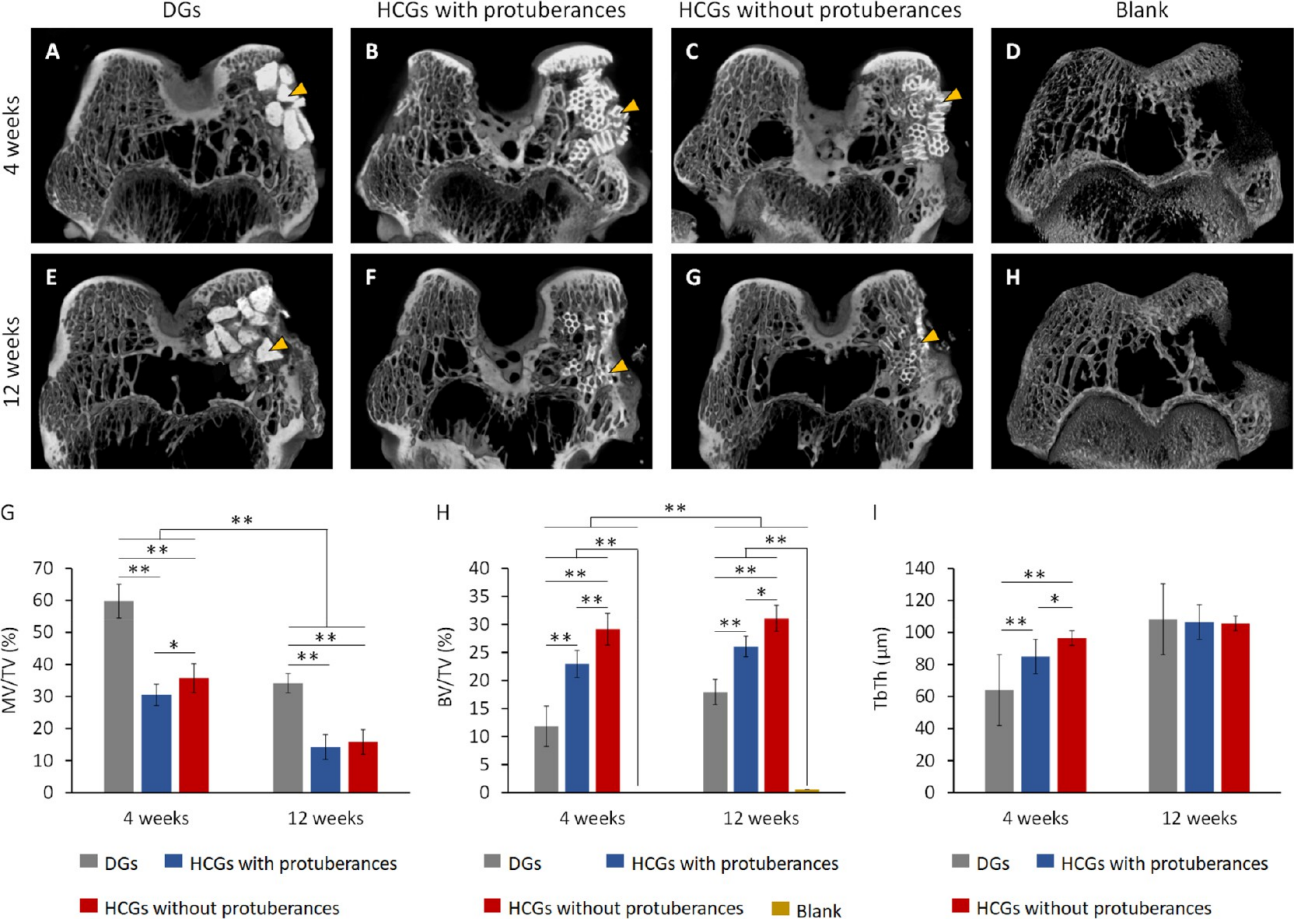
μ-CT images at (A–D) 4 and (E–H) 12 weeks after
